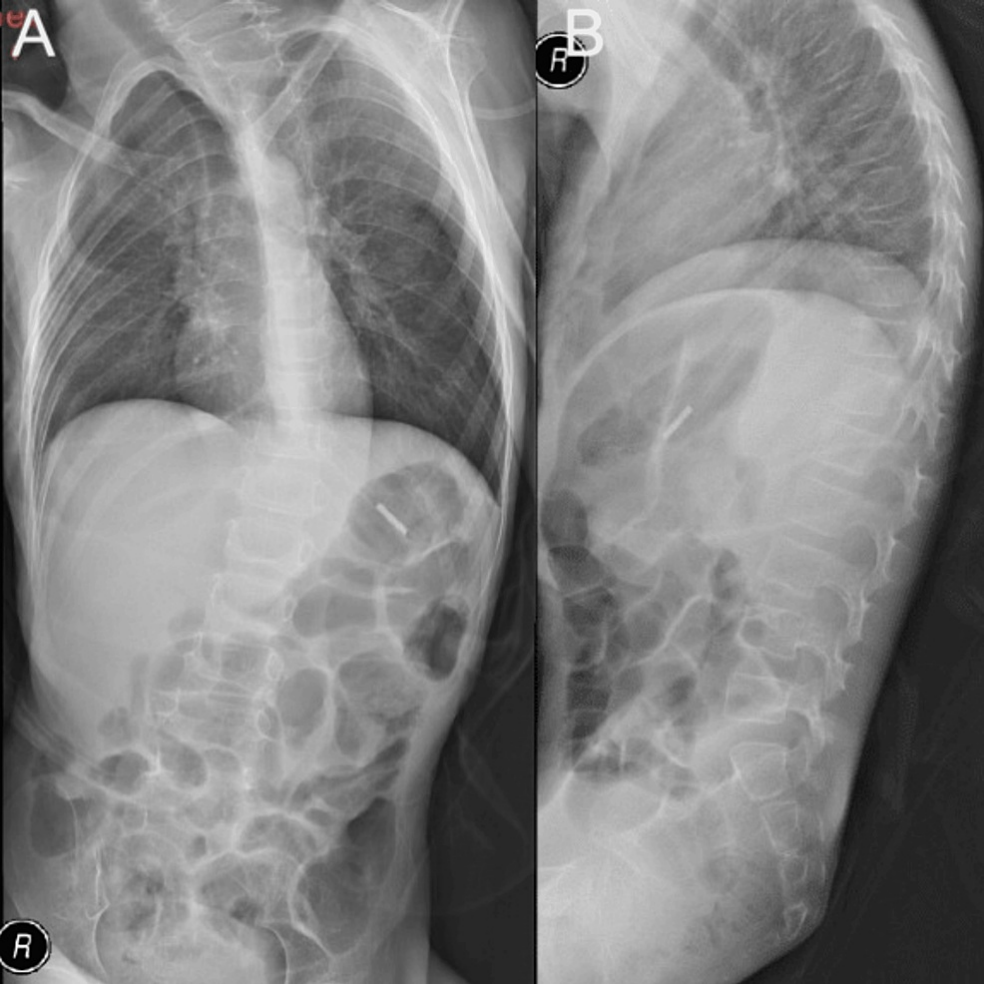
Supine AP X-ray (A) showing left-sided scoliosis with diffuse reduced bone density, and lateral X-ray from the side (B)
